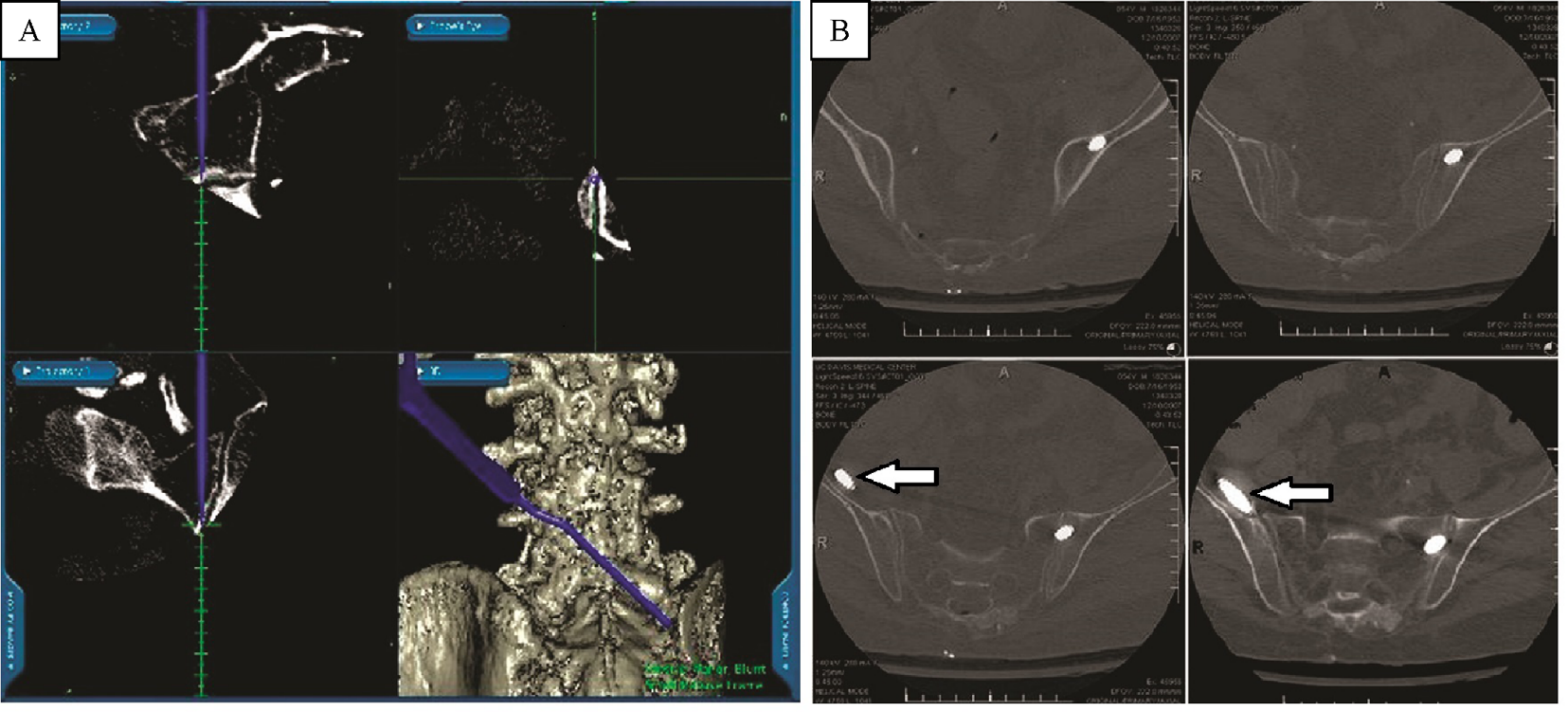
Biomechanial study constructs. A: Stealth IGS snapshot showing preoperative trajectory planning. Note the lack of inclusion of the right ilium in the CT field of view. B: Postoperative CTshowing axial images of malpositioned right SI screw placed under fluoroscopic guidance (indicated by arrows) compared with a properly positioned left sacroiliac screw placed via IGS. IGS: image guidance system.
